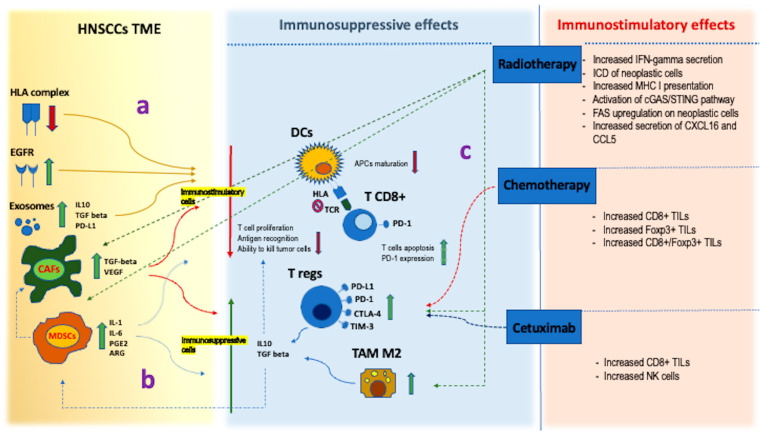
The degree of immunosuppression in posttreatment laryngeal SCC TME relies upon several factors fostering each other. SCC-related alterations of TME are responsible for a reduction of immune response by decreasing the activity of immune-stimulatory cells. Downregulation of the HLA complex impairs the process of antigen recognition by CD8+ T-cells [25]. Increase of tumor-derived exosomes alters TME toward an immunosuppressive fashion environment in many ways, including the inhibition of T-cells function and the modification of many several immunoregulatory factors such as PD-L1, IL-10, and TGF-beta [26]. EGFR is upregulated in most HNSCC, and activation of its downstream pathway hampers immune activation through several mechanisms, including downregulation of antigen presentation [27] (a). MDSCs and CAFs are typically increased in HNSCC TME, and they secrete a number of cytokines and soluble factors suppressing CD8+ T-cells, such as prostaglandins (PGE2), arginase (ARG), IL-1, IL-6, TGF-beta, and VEGF. MDSCs and CAFs exert immune suppressive roles both by decreasing the activity of immunostimulatory cells and recruiting immunosuppressive cells represented by Tregs and TAM M2. The latter produce immunosuppressive cytokines such as IL-10 and TGF-beta, which in turn further increase MDSCs within the TME and suppress T-cells functions [28,29,30,31,32] (b) T-regs are remarkably increased in TME of HNSCC and they show an upregulation of immune checkpoint molecules, namely PD-1, PD-L1, and CTLA-4 [33,34,35]. PD-1 expression is also increased on T CD8+ cells in HNSCCs [29,30]. On the other side, RT, CT, and Cetuximab are capable of both immunosuppressive and immunostimulatory effects. Radiotherapy contributes to the increase of MDSCs and CAFs, thus indirectly enforcing the immunosuppressive mechanisms described above [28]. At the same time, RT, CT, and Cetuximab increase Tregs in TME and favour the upregulation of PD-L1, PD-1, CTLA-4, and TIM-3 on the Tregs surface. Furthermore, RT recruits TAM M2, which, as already pointed out, fulfils an immunosuppressive activity [28] (c).
